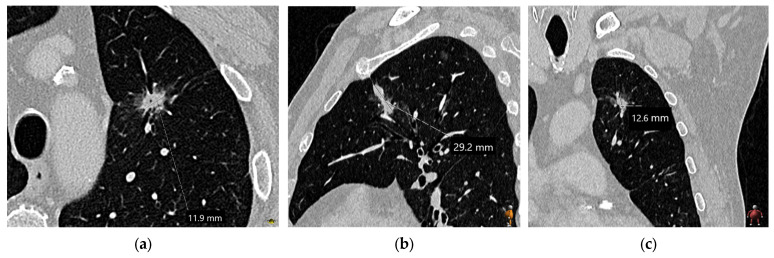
Measurement of solid nodule/mass diameter: The recommended diameter measurement of solid lung cancer on chest CT involves the use of the lung window setting (width 1500, level −600) with the measurement of the longest diameter in the axial (a), sagittal (b), and coronal planes (c). The longest measurement is used for staging; in this case, a sagittal diameter of 2.9 cm indicates the T1c stage. Only the nodule core should be measured, and spiculations should be excluded from the measurement. Measurements for pure ground-glass nodules follow the same principles as measurements for solid nodules.
